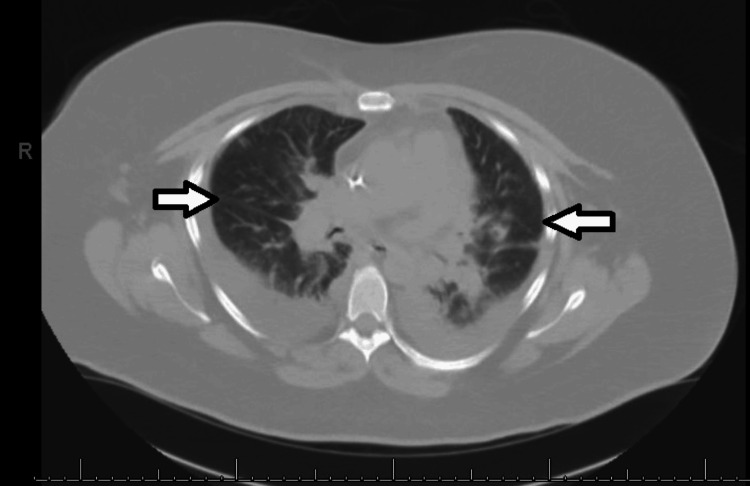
Computed tomography scan demonstrating patchy ground-glass opacities in bilateral lower lobes.
